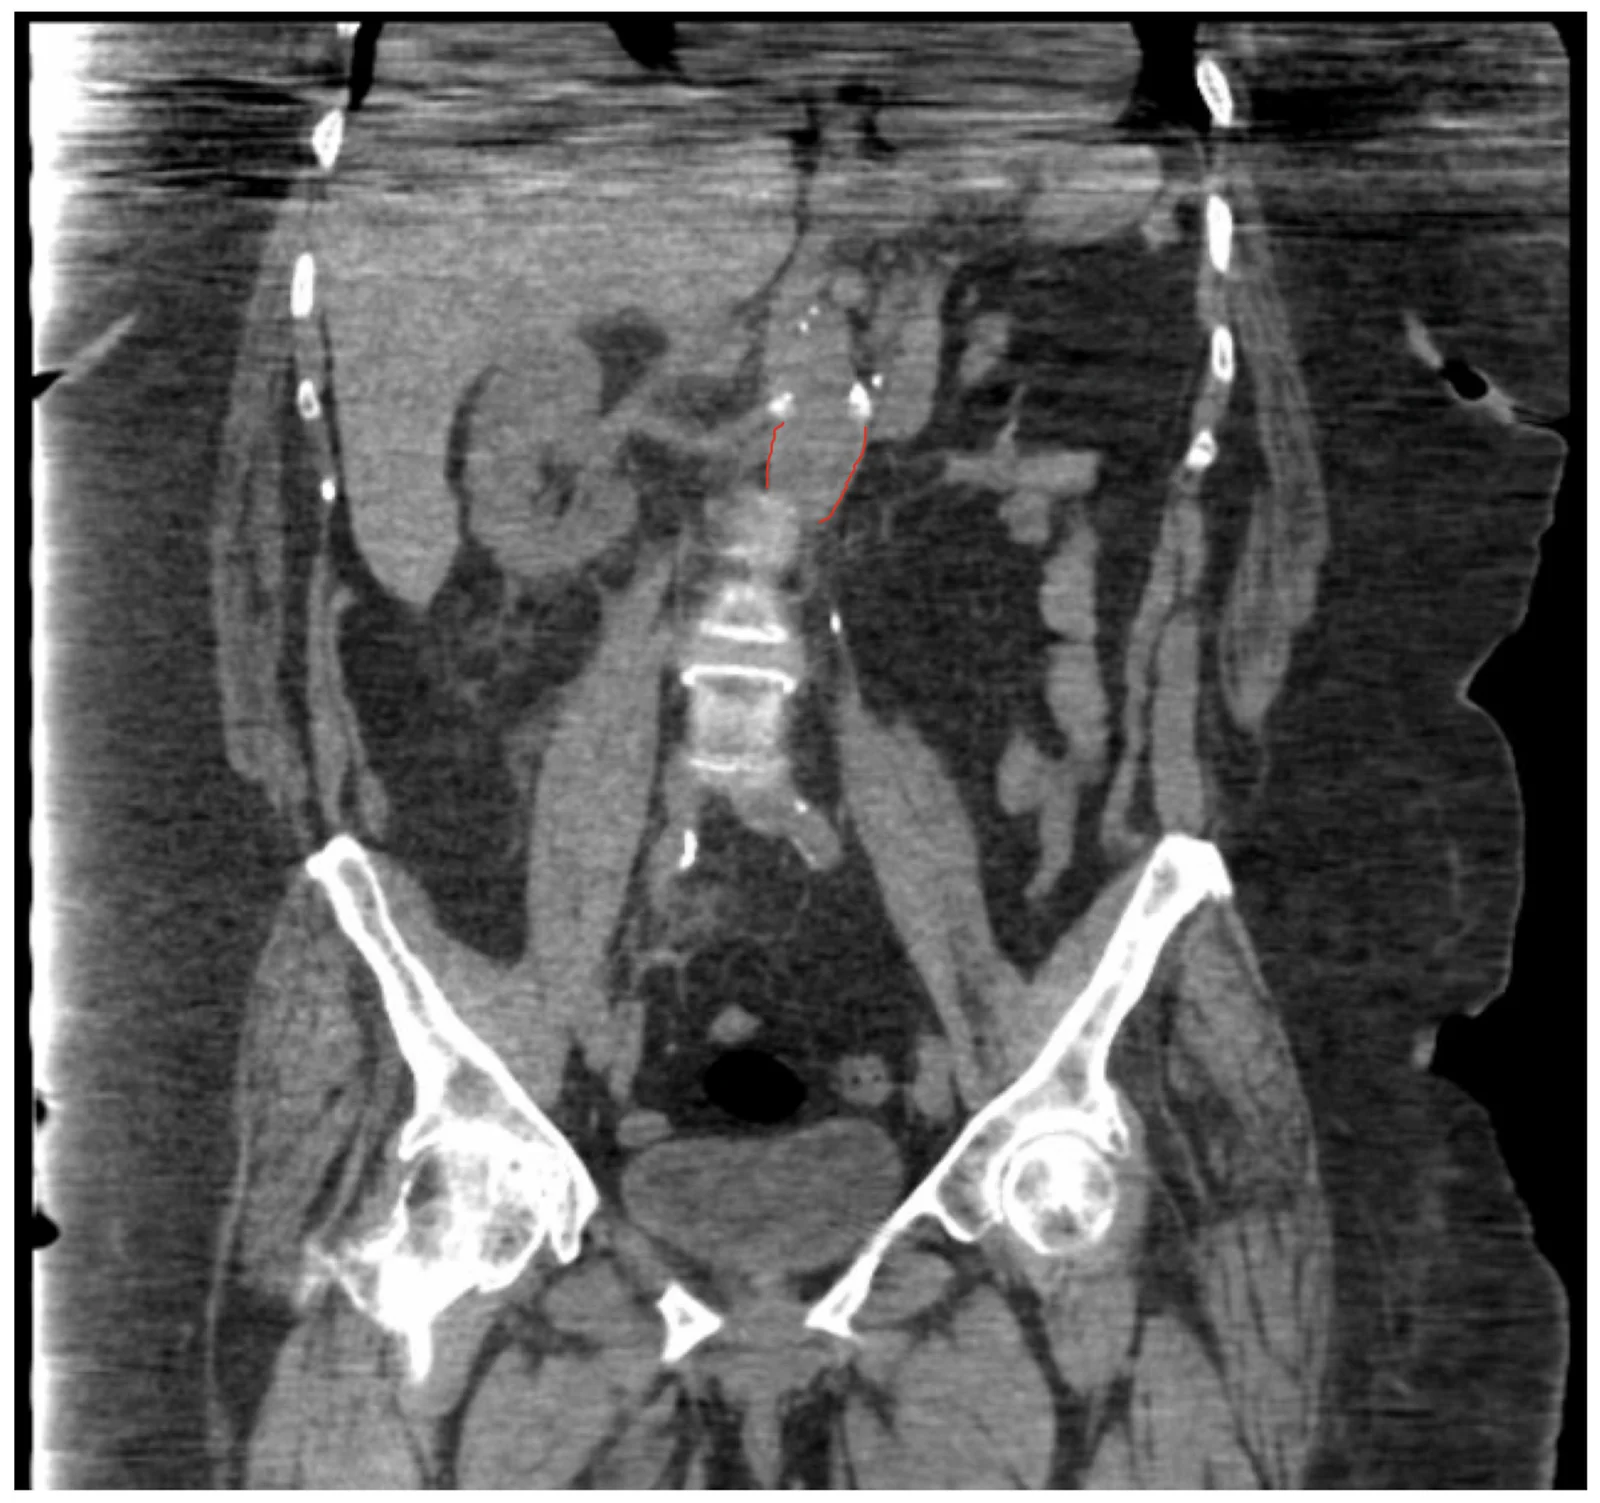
Postoperative noncontrast computed tomography (CT) demonstrating the aortic interposition graft, outlined in *red*.
